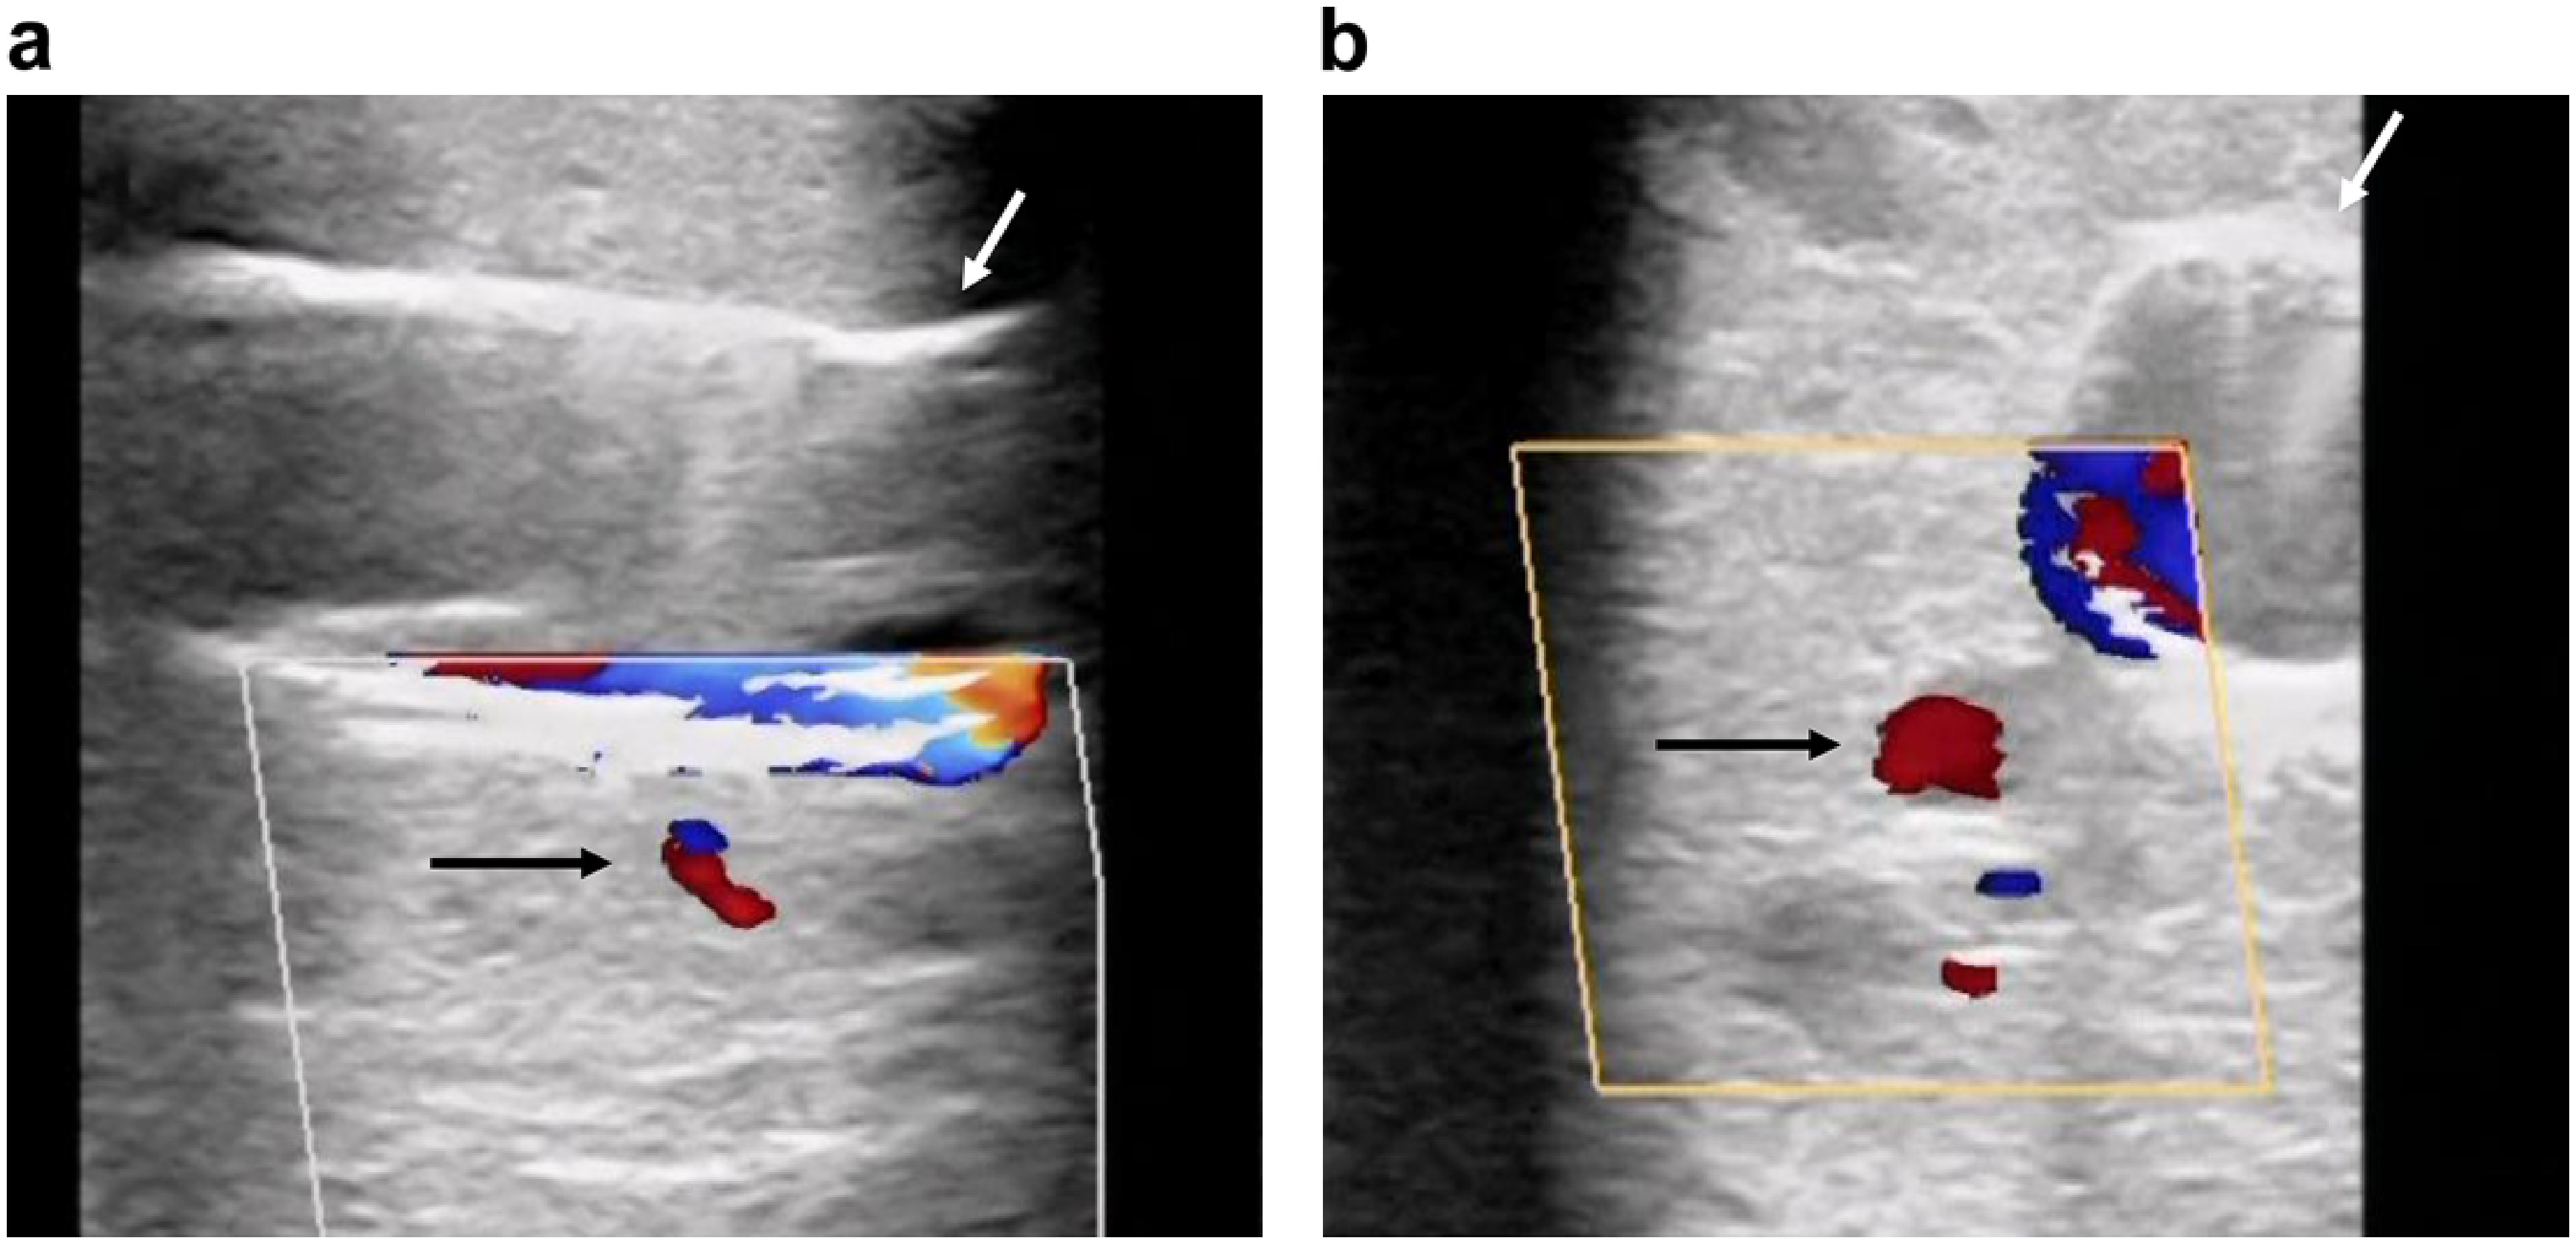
Fig. 2 Intraoperative epiaortic ultrasonography showing one of the target feeding vessels (black arrow) from the lumbar artery in the aneurysm next to the endograft (white arrow). (a) Long axis. (b) Short axis.
